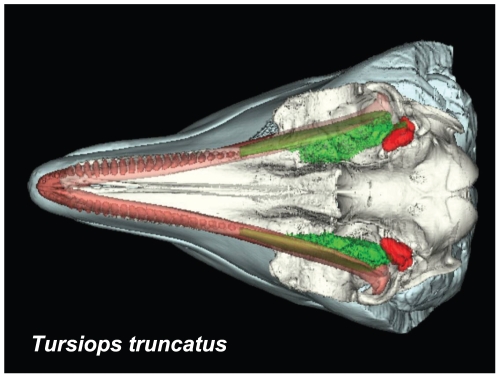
Ventral view of the sound reception anatomy in *Tursiops truncatus*, reconstructed from CT scans. Skin = cyan, skull = ivory, teeth and mandibles = salmon, mandibular teeth = salmon, mandibular fat bodies = green, TPC's = red.
